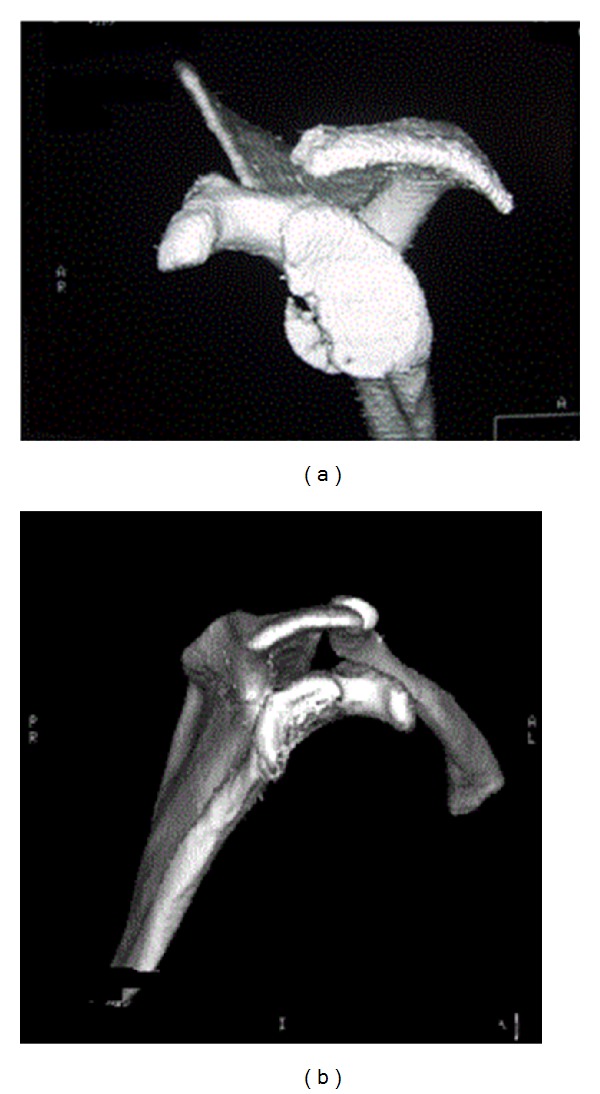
3-dimensional CT scans demonstrating (a) fragmentary bone loss and (b) attritional bone loss of the glenoid.
